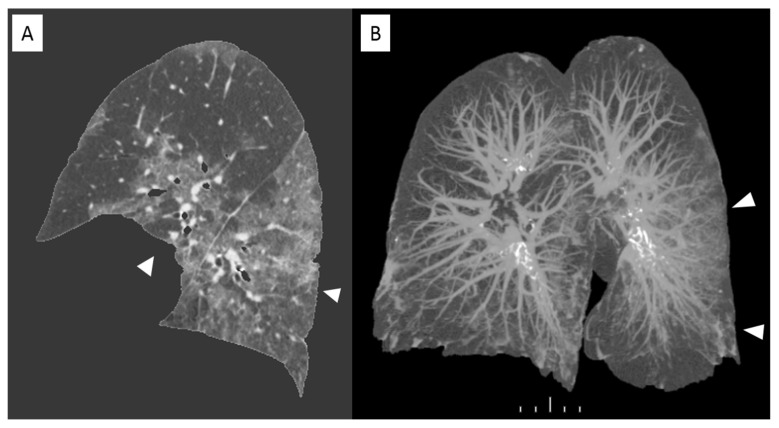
Sagittal view of a non-enhanced CT with software segmentation (A) showing extended GGOs (arrowheads). On the right the volume rendering recon of the same CT (B) highlighting the pulmonary vessels and the infected parenchyma hyperdense (arrowheads). in comparison with adjacent normal aerated lung.
